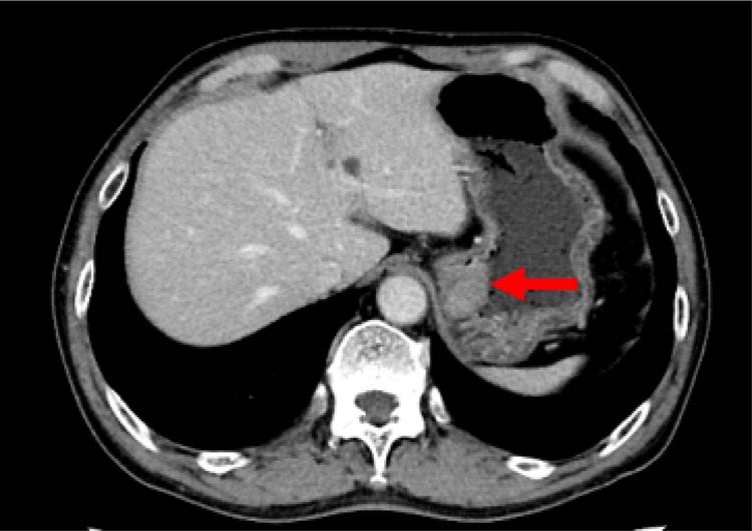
Contrast-enhanced CT showing the thickness of the gastric wall in the upper stomach (red arrow).
